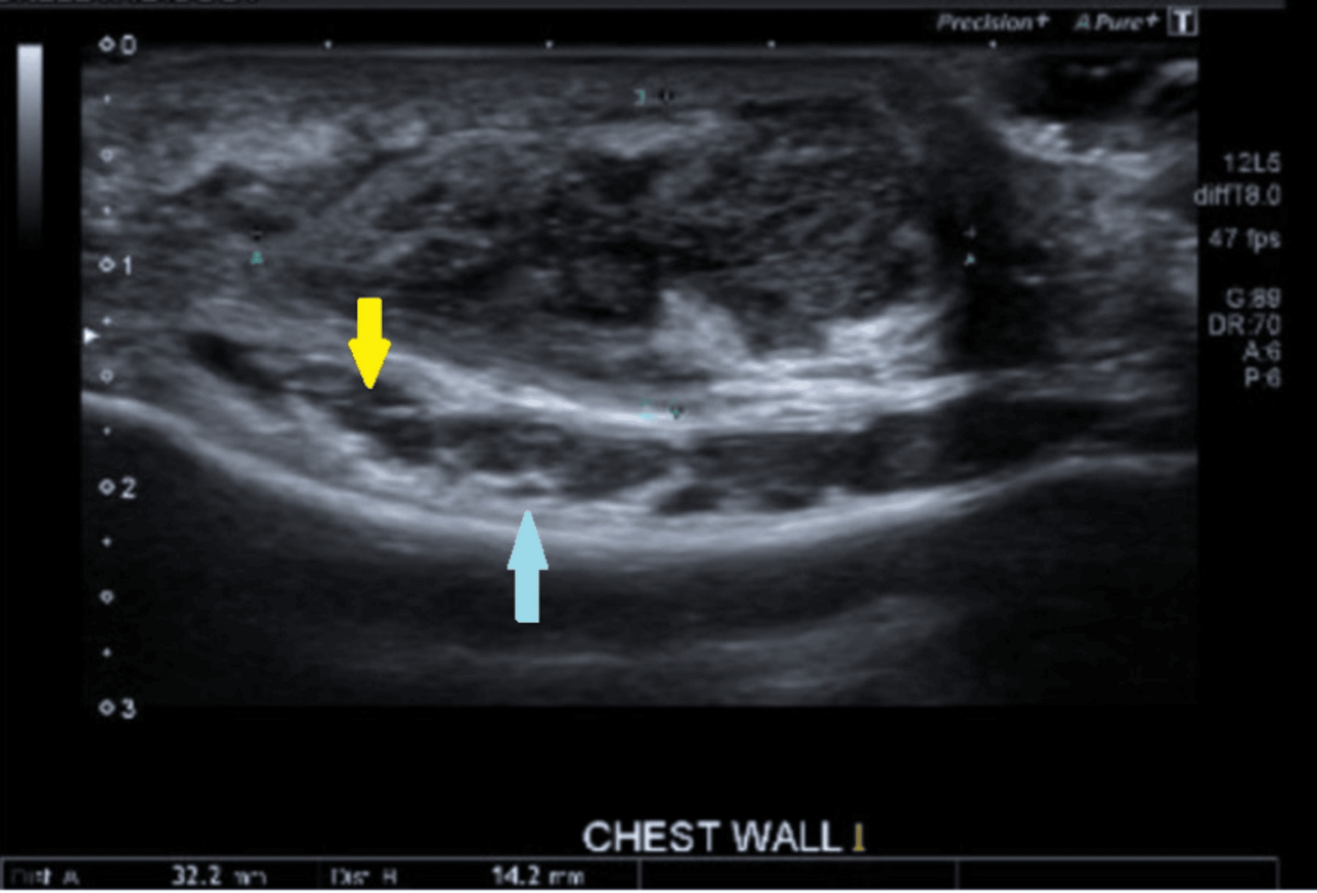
An ultrasound of the chest wall showed small collection 32 x 14 mm on the upper sternum (yellow arrow) with bony irregularity (blue arrow)
